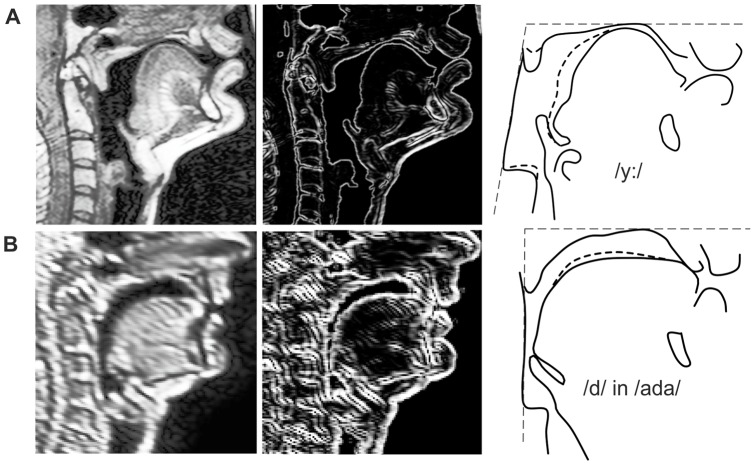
Example images from the two MRI corpora and their traced contours. A: Original midsagittal image of the vocal tract for the vowel /y:/ from the volumetric MRI corpus (left), the same image with enhanced edges (middle), and the traced contours (right). B: Same as A for an image of the real-time MRI corpus showing the consonant /d/ in /a/-context. The thick dashed lines in the traced images show the outline of the tongue side. The thin dashed lines indicate the angle of the rear pharyngeal wall with respect to the hard palate, which varies between the two corpora. The traced images were rotated for an identical orientation of the hard palate. (Figure modified from [17]).
